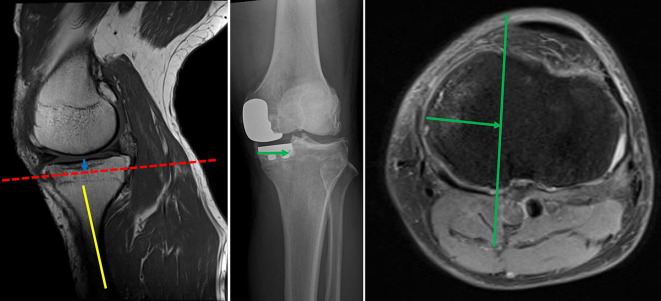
The tibial resection width (green arrow) measured from the anteroposterior image of the postoperative radiograph was projected onto the transverse plane containing the intersection of the virtual tibial cut line and the posterior tibial cortex, after which a line was drawn parallel to the medial or lateral intercondylar ridge (green line).
